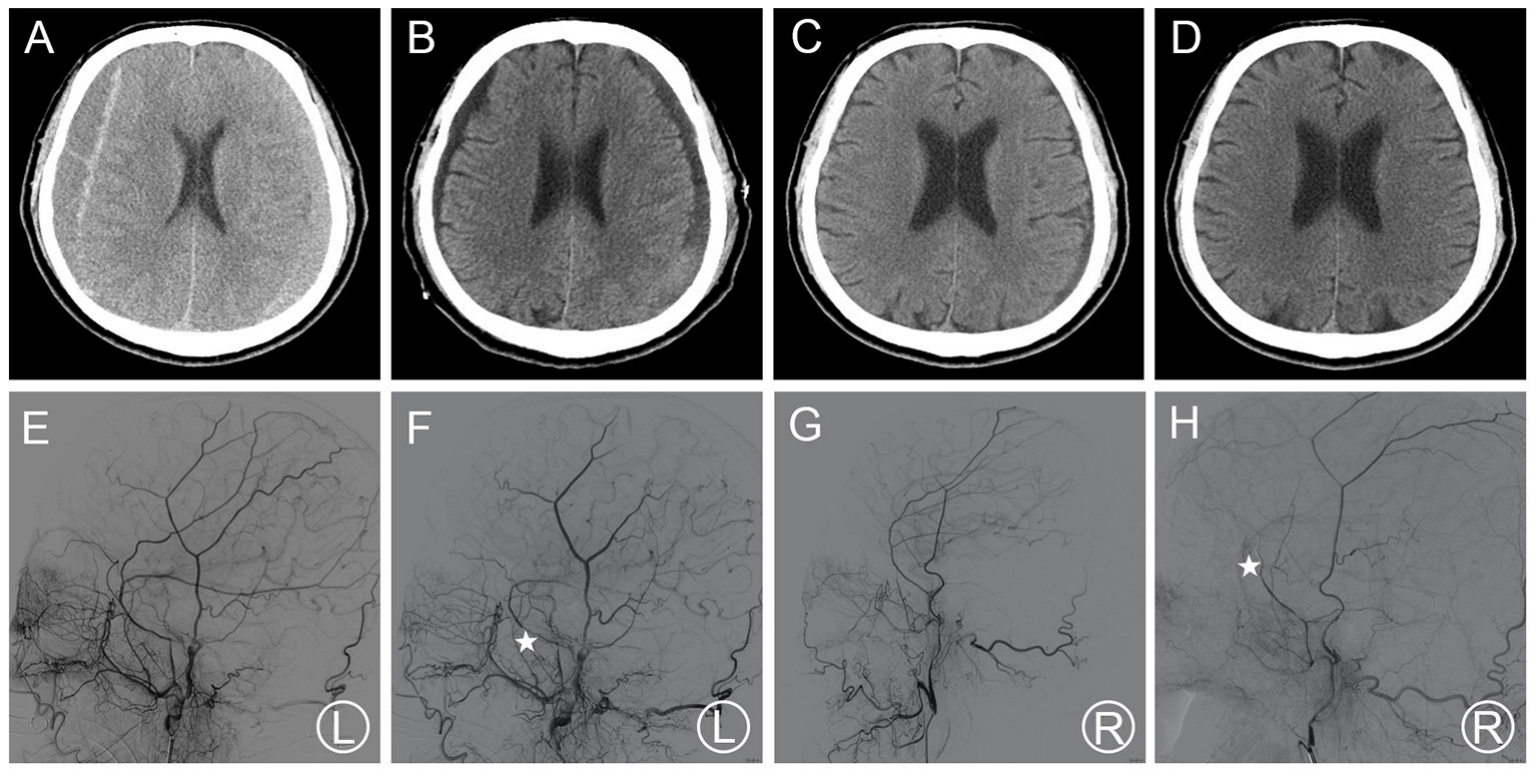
(A) Brain CT images of a hematoma in patient 1 before treatment. (B) Brain CT was repeated before the patient's discharge. (C,D) Follow-up brain CT was performed at 35 and 115 days. (E,F) Pre- and post-operative DSA presented left MMA and the left MMA disappeared (asterisk). (G,H) Pre- and post-operative DSA presented right MMA and the right MMA disappeared (asterisk). DSA, digital subtraction angiography; CT, computed tomography; MMA, middle meningeal artery.
